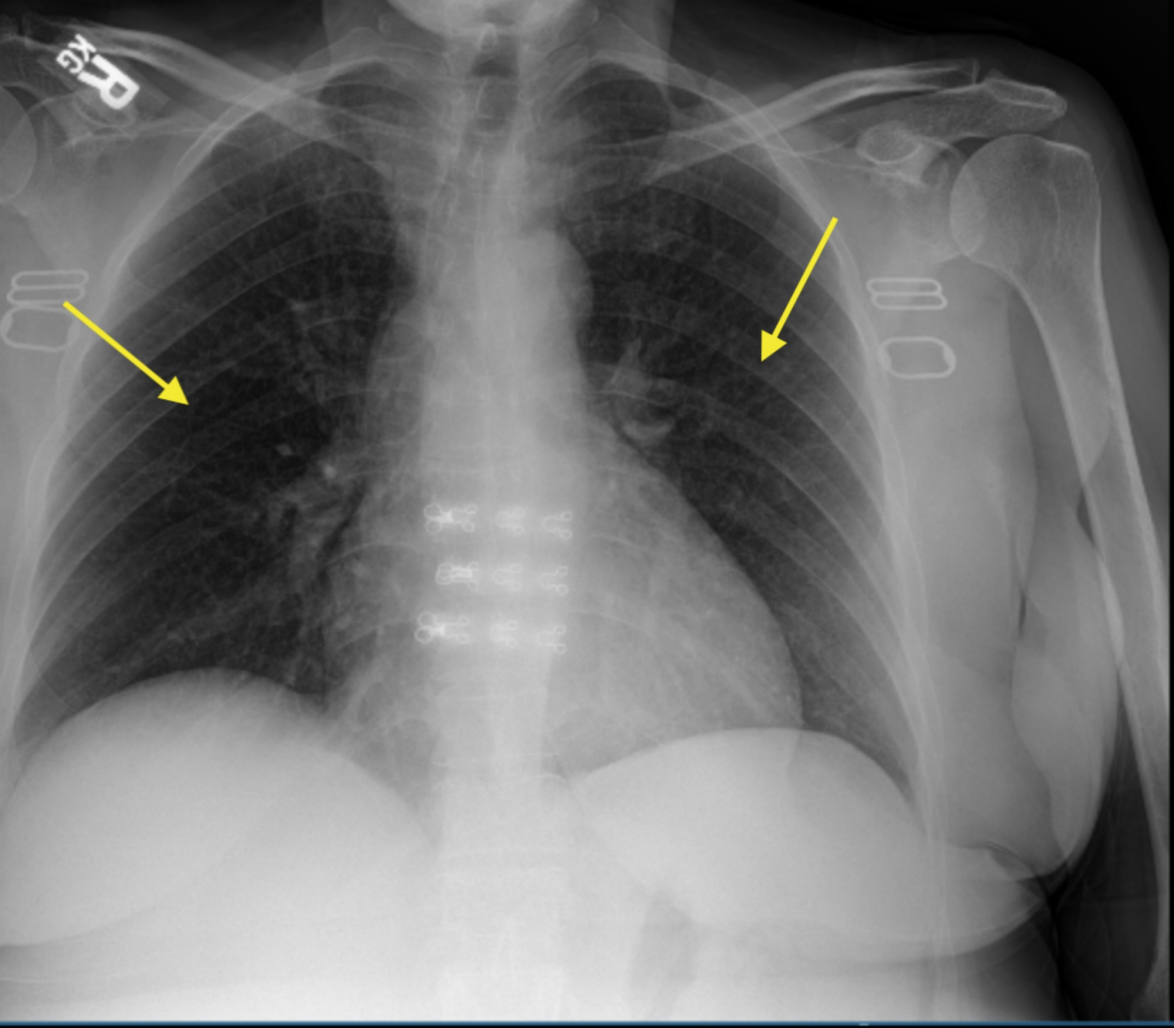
Chest X-ray showing no significant findings (yellow arrows)
